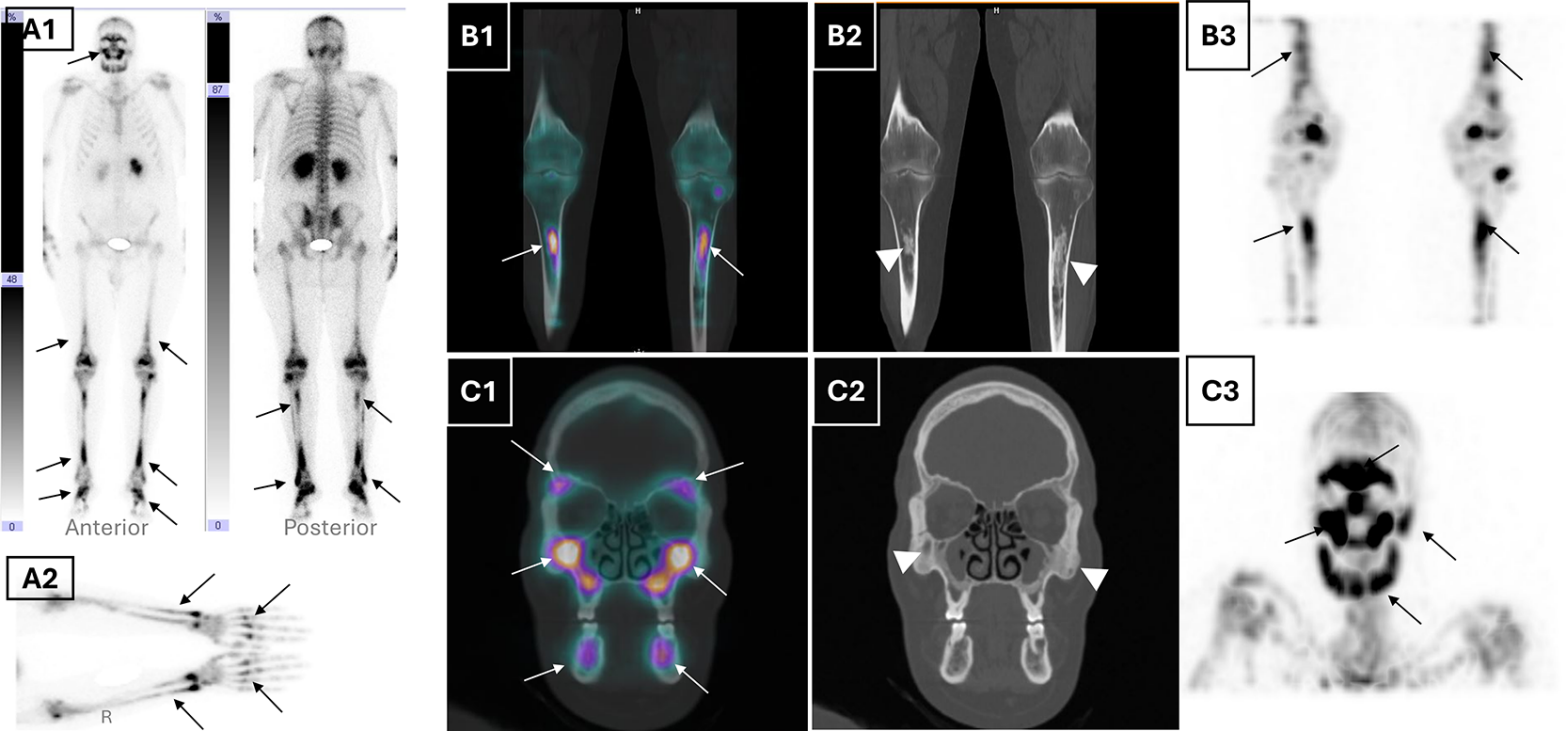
Multimodal nuclear bone imaging demonstrating typical skeletal and craniofacial involvement in ECD. (A1) Whole-body planar bone scintigraphy (anterior and posterior views) shows diffuse symmetric and bilateral metaphyseal-diaphyseal increased radiotracer uptake in the distal femora, tibiae, feet, and maxillofacial skeleton (black arrows), consistent with systemic osseous involvement. (B1) Coronal fused SPECT/CT and (B2) corresponding CT of the lower limbs show bilateral metaphyseal-diaphyseal uptake in the femurs and tibiae (white arrows), with associated cortical thickening and sclerosis (white arrowheads). (A2) Static planar view of the hands and forearms highlights pronounced tracer uptake in the metacarpals and distal radius/ulna (black arrows), supporting upper extremity involvement, which is less commonly reported in Erdheim-Chester disease. (B3) Coronal MIP SPECT image confirms symmetrical uptake in the long bones of the lower extremities (black arrows). (C1) Coronal fused SPECT/CT and (C2) CT of the maxillofacial bones highlight tracer accumulation in the maxillae, zygomatic arches, and mandibles (white arrows) and corresponding sclerotic changes (white arrowheads). (C3) MIP SPECT image of the skull reveals diffuse symmetric craniofacial tracer uptake, notably involving the orbitomaxillary complex and mandible (black arrows). Abbreviations:
